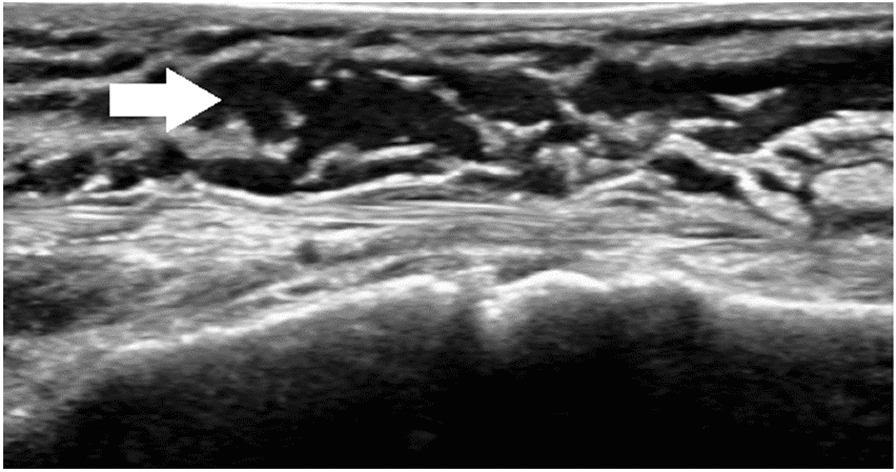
Subcutaneous interstitial edema (white arrow) and absence of Doppler flow were noted on ultrasound
